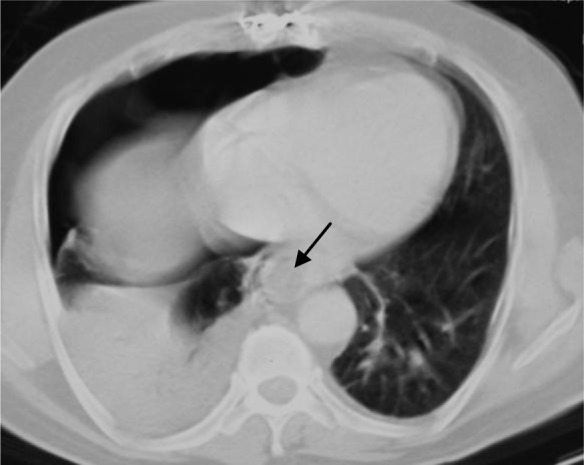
Chest CT demonstrates right side hydropneumothorax and impacted foreign body in the esophagus (arrow)
